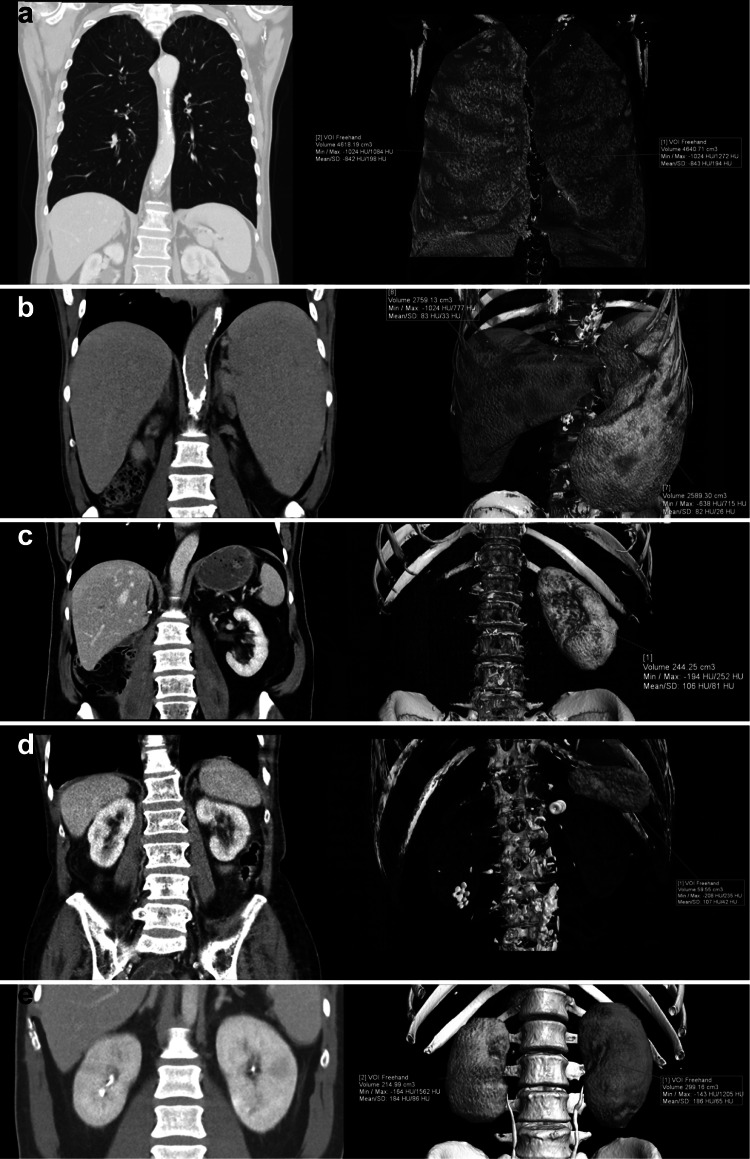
(a) Exemplary case of a 48-year-old patient with known obstructive lung disease and emphysema. The CT examination was scheduled with the suspicion of a rectal bleeding. The manual contour segmentation with shape interpolation yielded a left lung volume of 4640 ml and a right lung volume of 4618 ml. NeuronX calculated a left lung volume of 4787 ml (+ 147 ml) and a right lung volume of 4760 ml (+ 142 ml). (b) Exemplary case of a 62-year-old patient with a myeloproliferative disease and a known hepato- and splenomegaly. The CT examination was scheduled due to a suspected spleen rupture. The manual contour segmentation with shape interpolation yielded a liver volume of 2759 ml and a spleen volume of 2589 ml. NeuronX calculated a liver volume of 2812 ml (+ 53 ml) and a spleen volume of 2608 (+ 19 ml). (c) Exemplary case of a 67-year-old patient with a right kidney resection due to a sarcoma. The CT examination was scheduled due to a suspected bleeding in the postoperative bed. The manual contour segmentation with shape interpolation yielded a left kidney volume of 244 ml. NeuronX calculated a left kidney volume of 267 ml (+ 23 ml). NeuronX did not calculate any values for the missing right kidney as the 3D landmark detection algorithm did not find the object in the 3D space. The case was not included in the final analysis as it did not fulfill the inclusion criteria. (d) Exemplary case of a 45-year-old patient with a suspected intestinal bleeding. The manual contour segmentation with shape interpolation yielded a spleen volume of 60 ml. NeuronX calculated a spleen volume of 66 ml (+ 6 ml). e Exemplary case of a 32-year-old patient with a suspected intestinal bleeding. The examination revealed a pyelonephritis affecting both kidneys. The manual contour segmentation with shape interpolation yielded a right sided kidney volume of 215 ml and a left sided kidney volume of 299 ml. NeuronX calculated a right sided kidney volume of 224 ml (+ 9 ml) and a left sided kidney volume of 315 ml (+ 16 ml)
